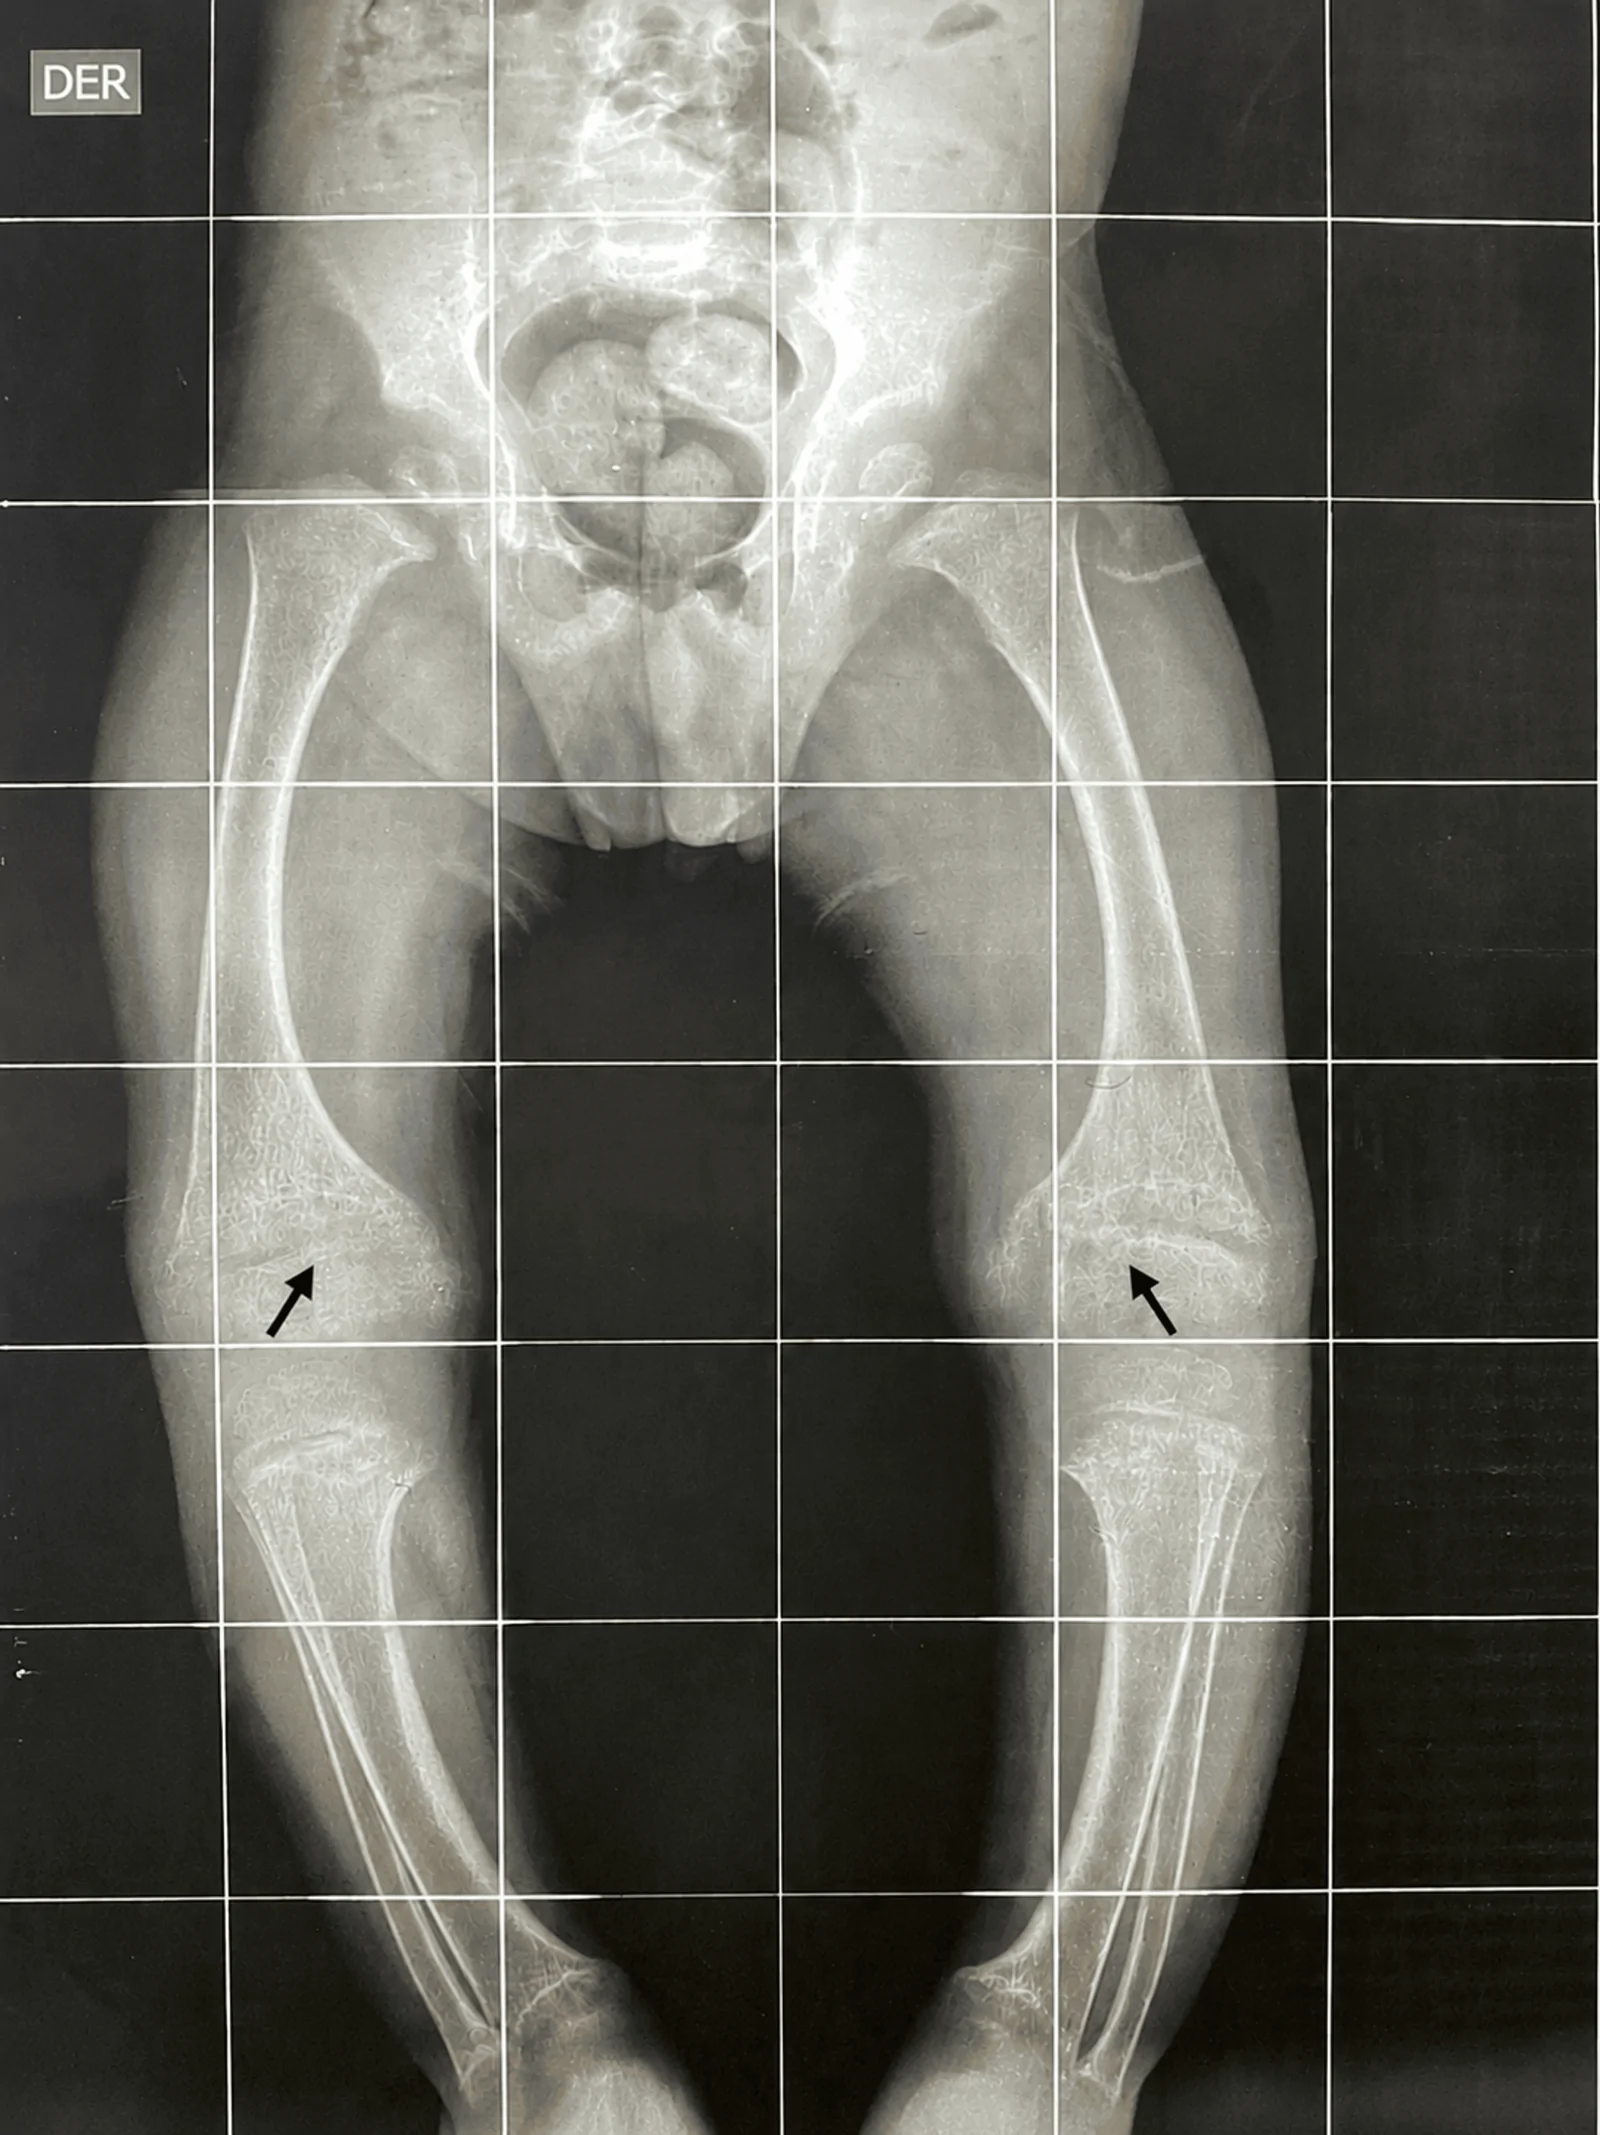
Lower limb radiograph DER: right Anteroposterior radiograph of the lower limbs demonstrating bilateral genu varum, with arrows indicating metaphyseal widening and irregular, frayed metaphyseal margins, characteristic findings of active rickets. The radiological severity corresponds to a Thacher score of 2.0 and is consistent with impaired bone mineralization secondary to renal phosphate wasting
